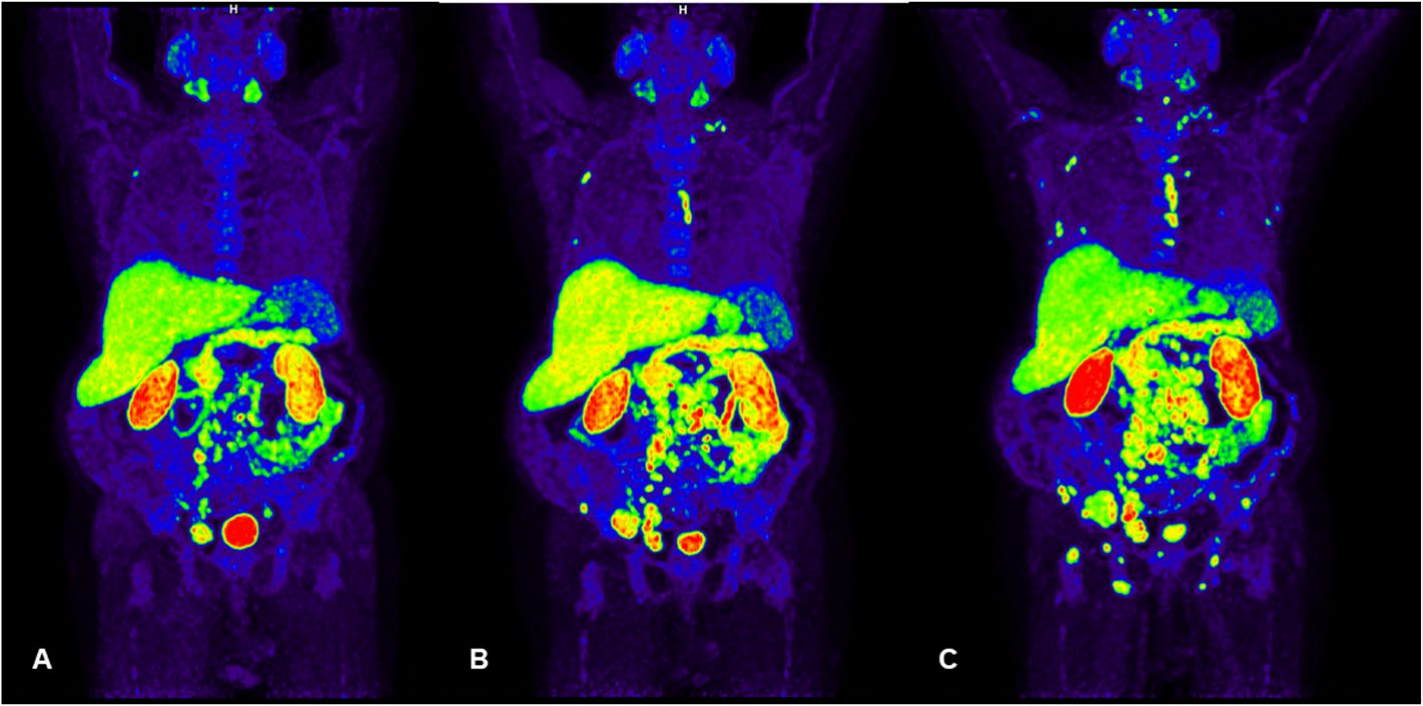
Baseline [18F]F-choline maximum intensity projection image (A) showed multiple skeletal and lymph node uptakes. Restaging [18F]F-choline maximum intensity projection images, performed 9 (B) and 11 (C) months after starting ADT respectively, showed a progressive increase of the bone and lymph node pathological uptakes (i.e. SUVmax progressively raising from 11.4 to 20.0 to 31.1 on L3 bone lesion)
